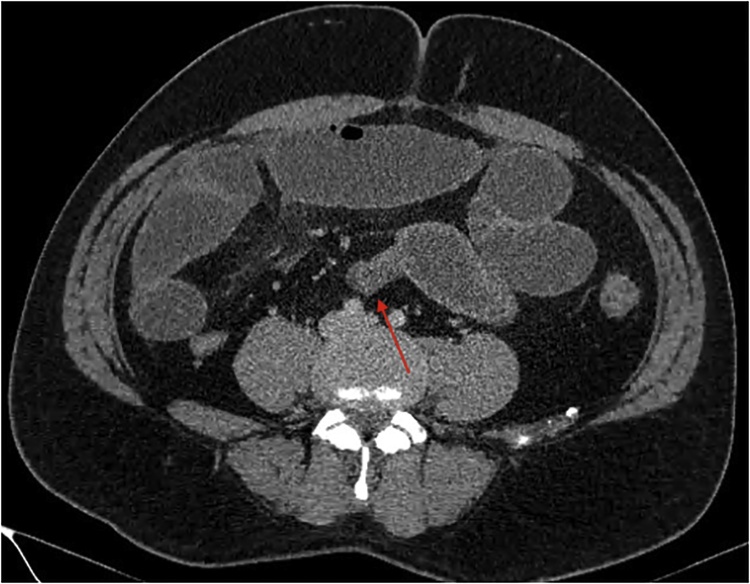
Axial view of the CT abdomen and pelvis showing transition point of the SBO with the angulation of the small bowel suggesting a kink.
